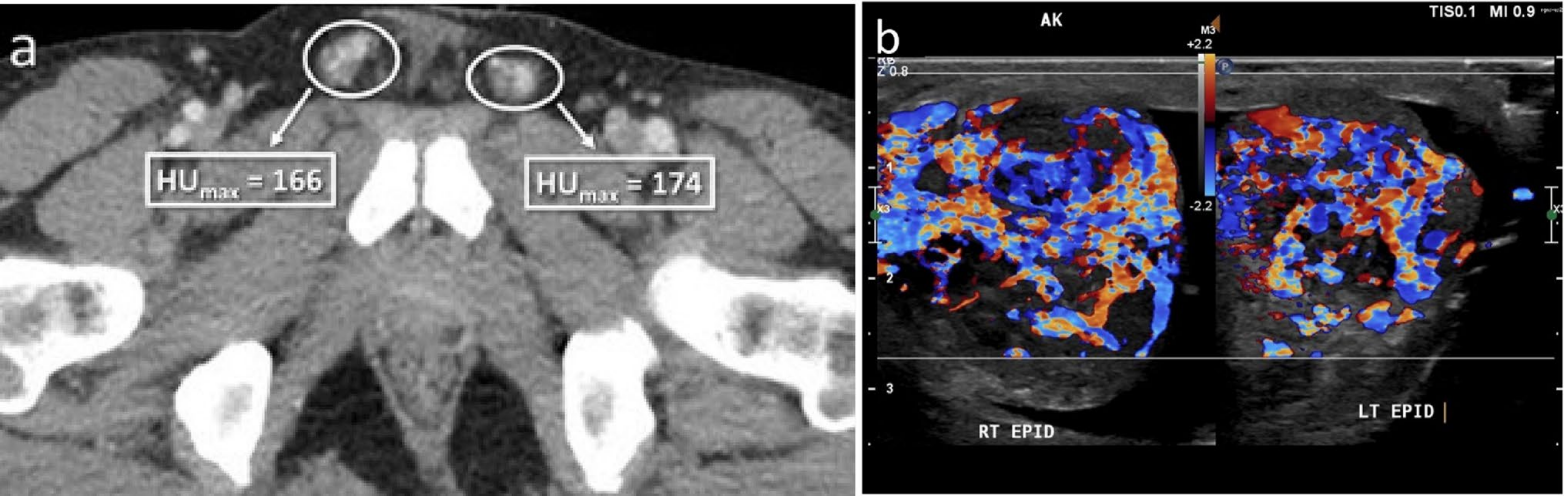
35-year-old patient with scrotal swelling, adenopathy, and elevated ESR. Quantitative analysis showed high bilateral HUmax, but differential enhancement of only 5% (a). All readers reported bilateral cord abnormalities with hyperemia and inflammatory change visible at CECT. Reader confidence ranged from 3 to 5 on a 5-point Likert scale. Color Doppler ultrasound confirmed bilateral epididymo-orchitis (b). Cord abnormalities were not appreciated at the prospective CT read
